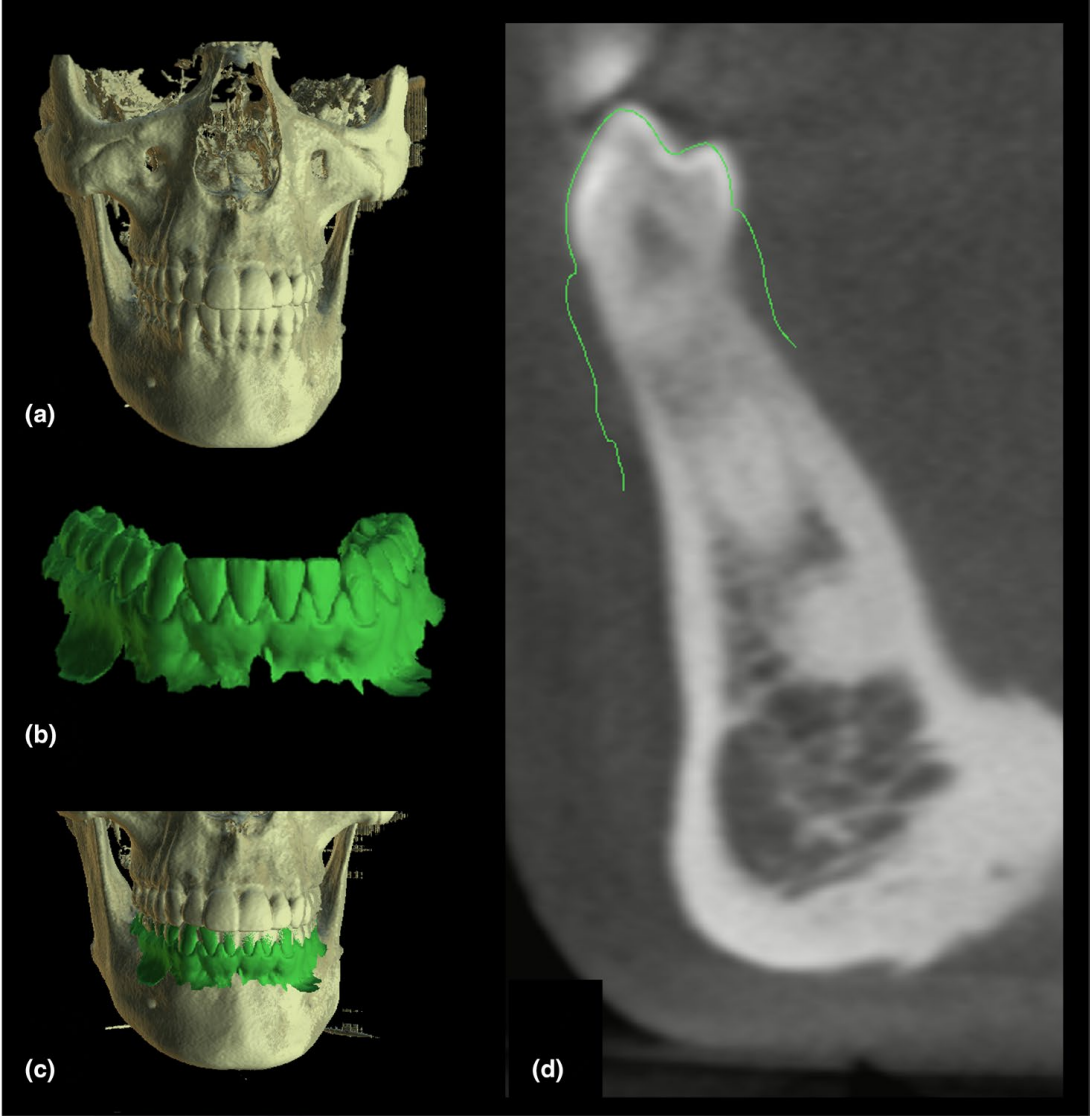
Integration of cone‐beam computed tomography (CBCT) (a) and intraoral scanning (b) for delineation of buccal gingival contours. The fused dataset is shown in (c), while the cross‐sectional view in (d) highlights the buccal gingival contours, indicated by the green line.
